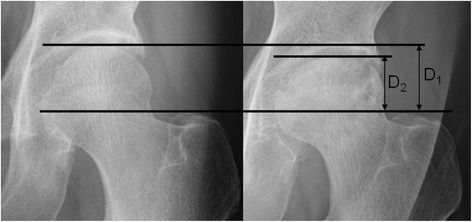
The progression of collapse of the femoral head of NOFH. The progression of collapse (D_1_-D_2_) before administration (*left*) and at every follow-up (*right*) using anteroposterior radiographs. The baseline is the top of the greater trochanter of the femur
